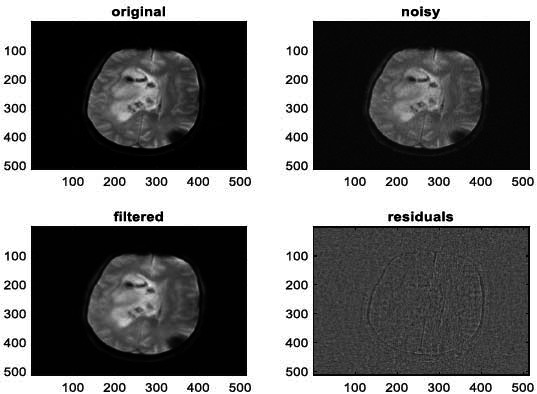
The first example of MRI Denoising by applying the proposed image denoising technique.
